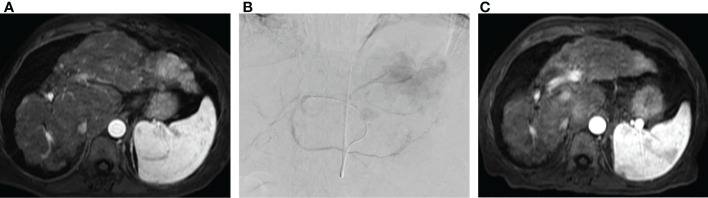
Radioembolization as salvage therapy. (A) Pre-procedure MRI in patient with metastatic colorectal cancer to the liver following three lines of chemotherapy demonstrating multifocal metastatic disease involving the left hepatic lobe. (B) Transradial radioembolization of the left hepatic lobe with Yttrium-90 resin microspheres *via* a replaced left hepatic artery arising from a left gastric artery. (C) Post procedure MRI with interval reduction in size and enhancement of left hepatic lobe tumor.
